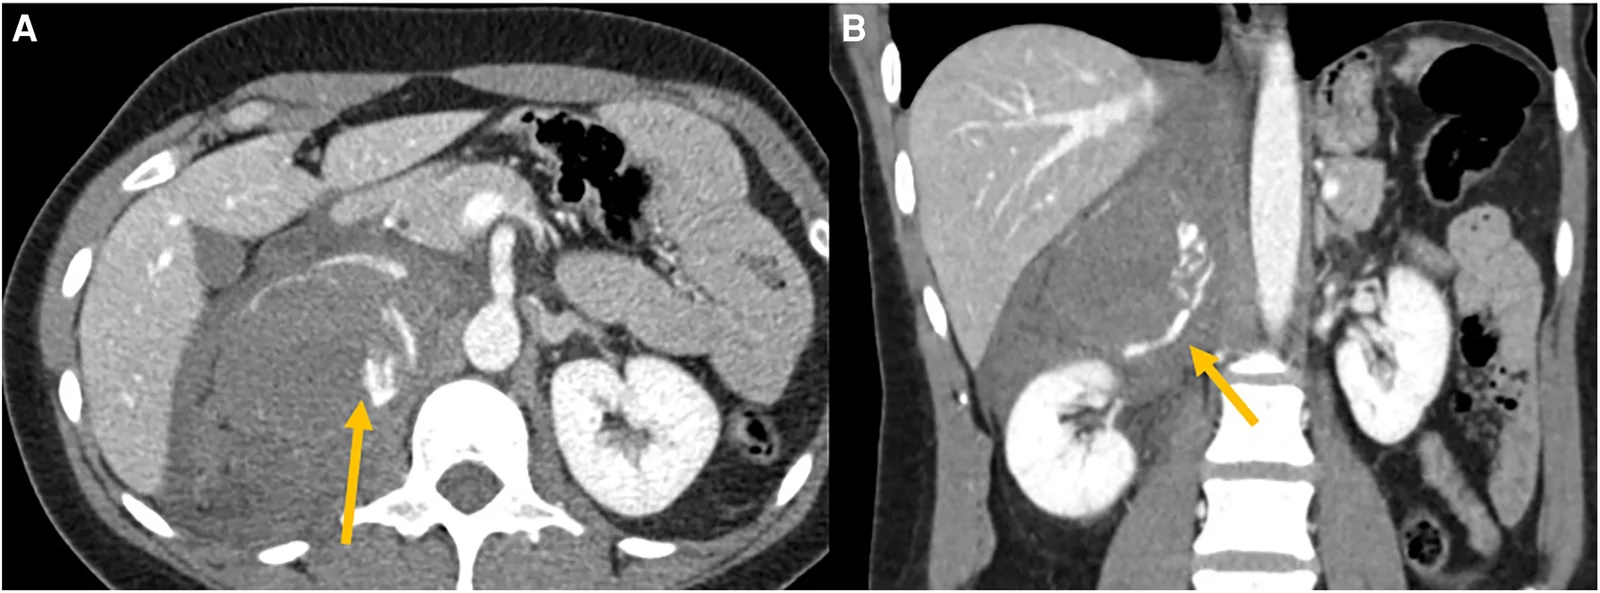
Contrast-enhanced computed tomography (CT) demonstrating a large right adrenal hemorrhage (AH). (A) Axial image showing a 5.4 × 6.9 cm right AH with acute blood products (arrow). (B) Coronal image demonstrating acute blood products on the adrenal bed (arrow).
